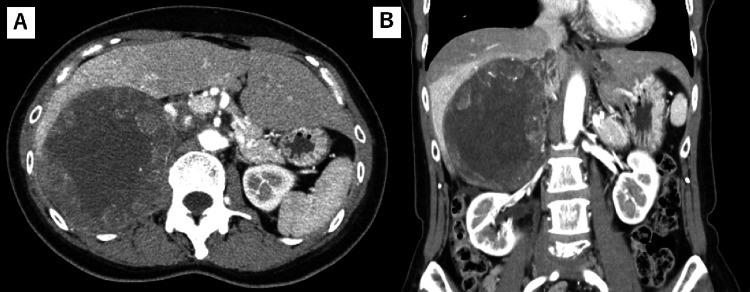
Adrenal gland tumor images before the adrenalectomy Right adrenal tumor images were obtained just before the adrenalectomy using enhanced CT in the transverse plane (A) and the coronal plane (B).
